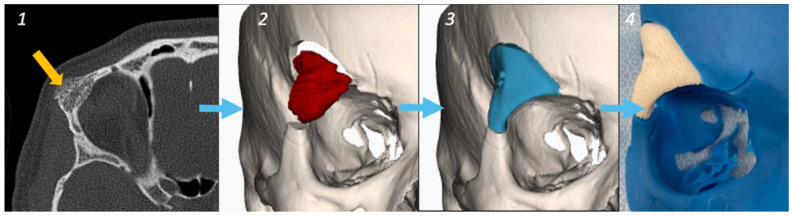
Planning, case 1. (1): Axial plane CT image of the supra-orbital region showing an extensive intra-osseous lesion (yellow arrow). (2): Digital segmentation of the lesion and planning of the resection margins. (3): Virtual design of the dimensions of the PSI. (4): Manufacturing and delivery of the implant.
